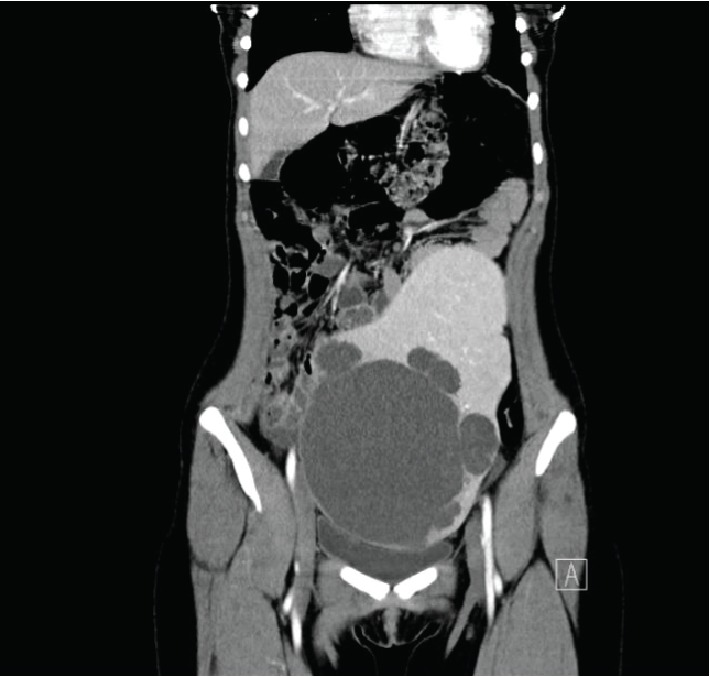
Coronal plane CT image of large intra-abdominal wandering spleen with cystic masses.
